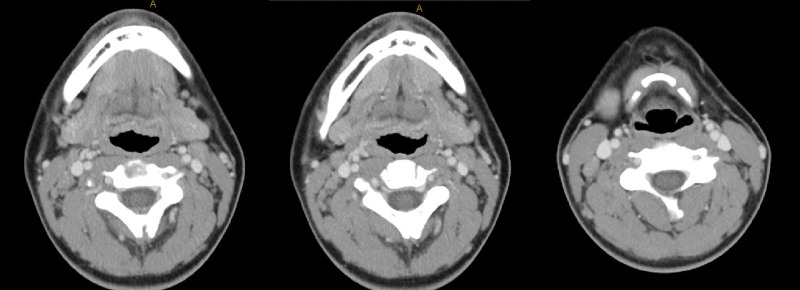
Neck CT. Reactive lymph nodes are observed in the neck level II–III, but no pathological findings are present. CT = computed tomography.
